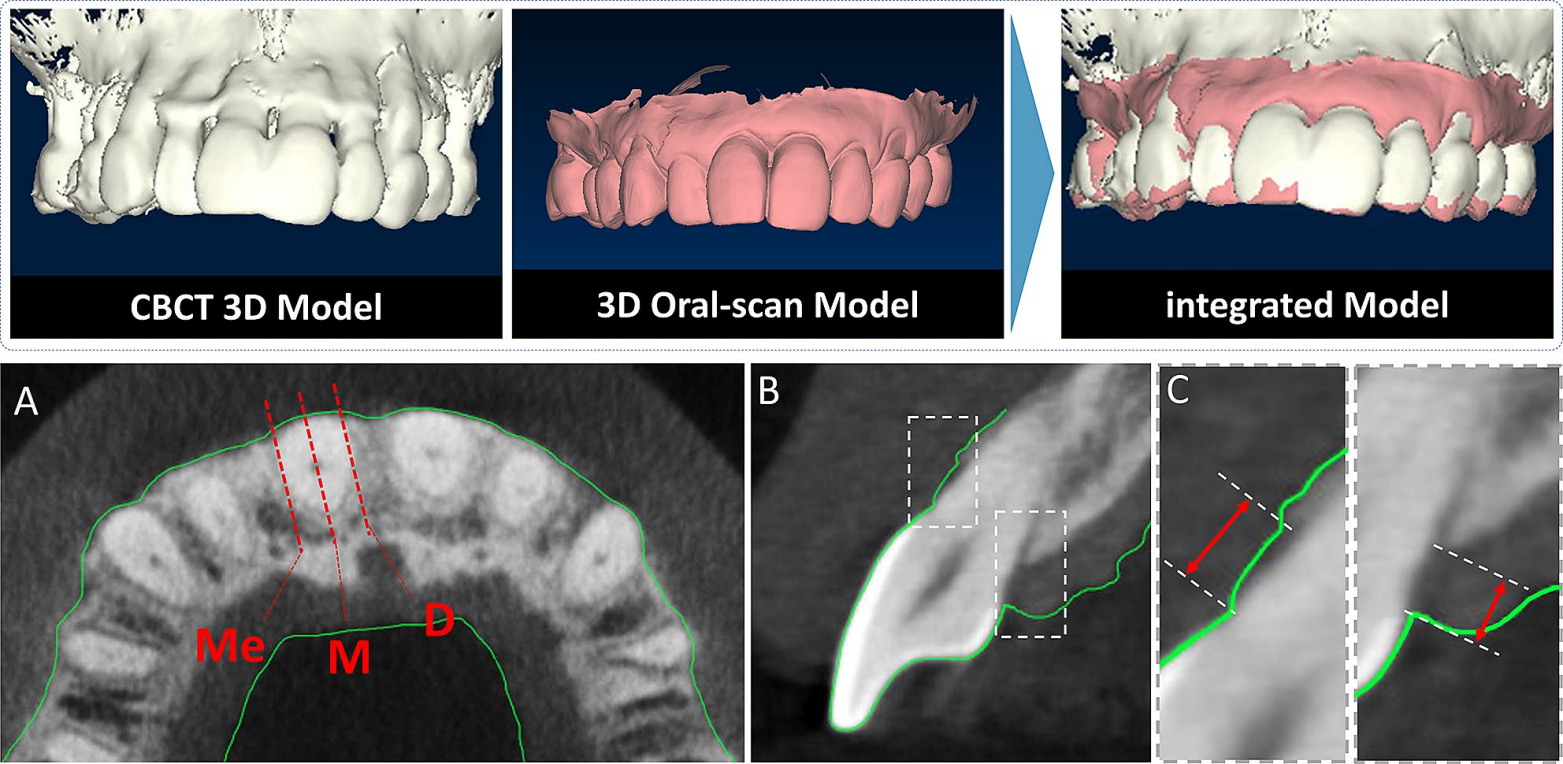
Cone-beam computed tomography (CBCT) images were acquired and integrated with an oral-scan model using 3D reconstruction software (OnDemand3D; Cybermed, Seoul, South Korea, https://www.ondemand3d.com/). The supra-alveolar gingival dimension (GD) was measured. (A) Axial image showing the positions selected for vertical cross-sectioning (mesial, middle, and distal). (B) Cross-sectional image of the dentogingival unit for GD measurement. (C) GD was defined as the distance between the most-coronal point of the marginal gingiva and the underlying alveolar bone.
